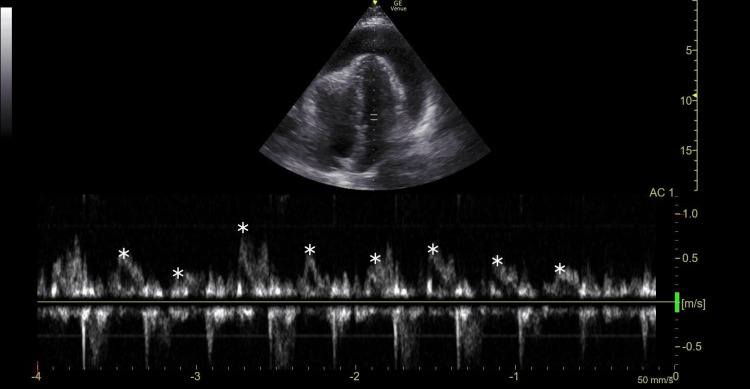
Apical four-chamber view with pulsed wave Doppler imaging demonstrating respiratory-phasic velocity changes of the mitral valve inflow velocities (*)
